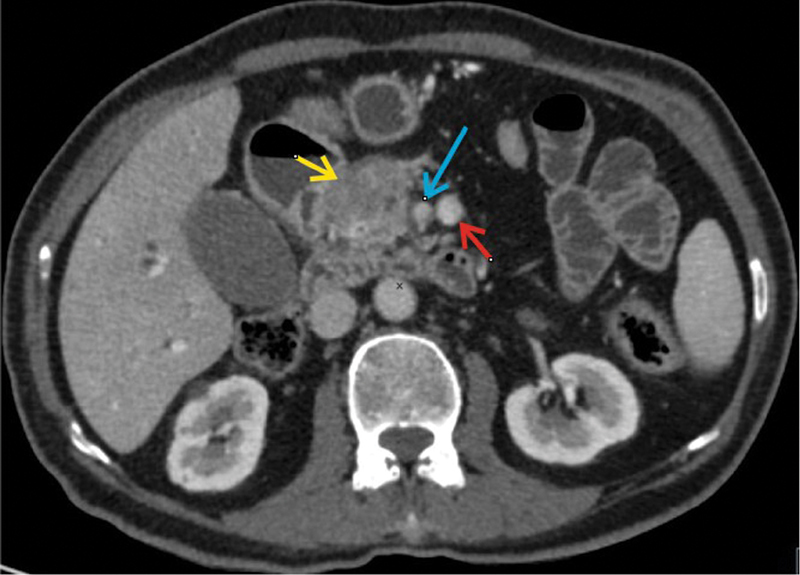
Axial contrast enhanced computed tomography scan image showing mass in the head of pancreas (yellow arrow) and its relation with superior mesenteric artery (blue arrow) and superior mesenteric vein (red arrow).
